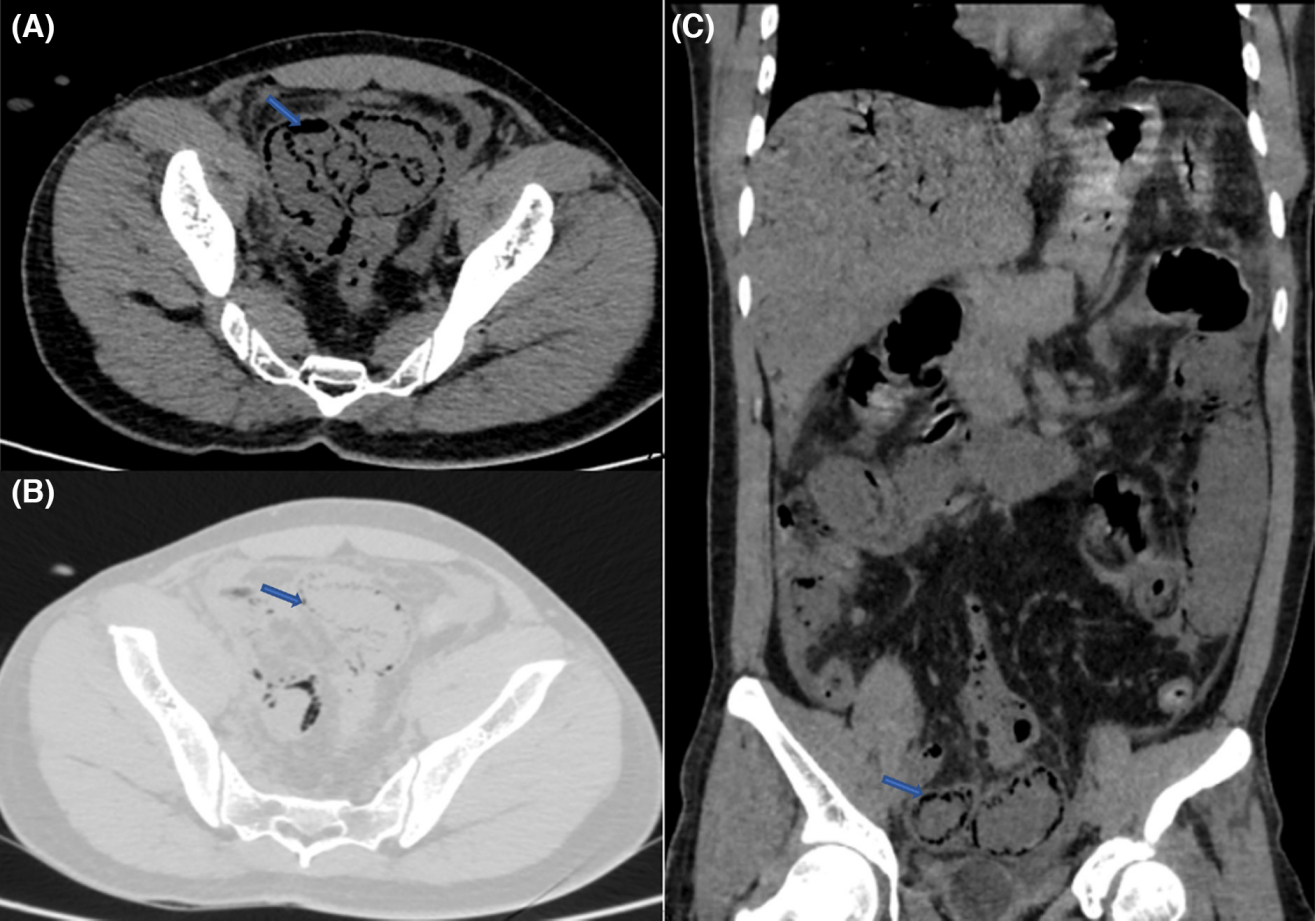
(A): axial noncontrast (soft tissue window), (B): axial noncontrast (lung window), and (C): coronal noncontrast CT images showing thinning of bowel walls in the pelvic region (probably small bowel loops) with multiple round cystic air attenuating areas within the bowel wall (arrows) demonstrating pneumatosis intestinalis.
